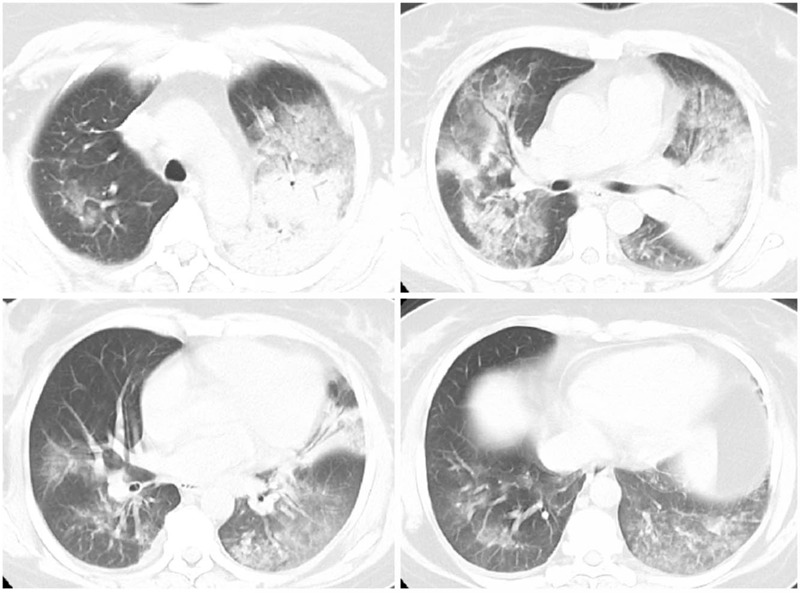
CT Scan of the female patient on admission revealed multiple patchy consolidation shadows and air bronchgram in bilateral lungs. CT = computed tomography.
